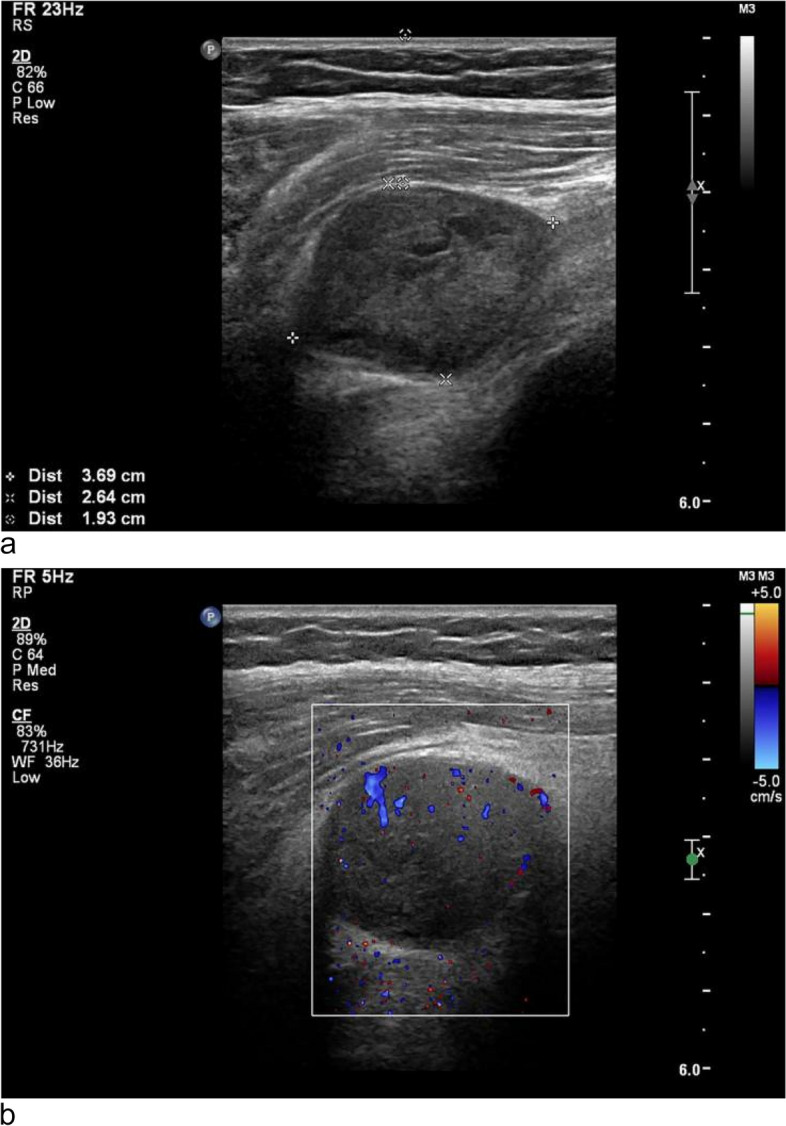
Ultrasound shows a solid mass in the deep muscle of right thigh suspiciously originating from the nerve. a The mass is located 1.9 cm away from the thigh surface. b Doppler flow can be detected in the mass
